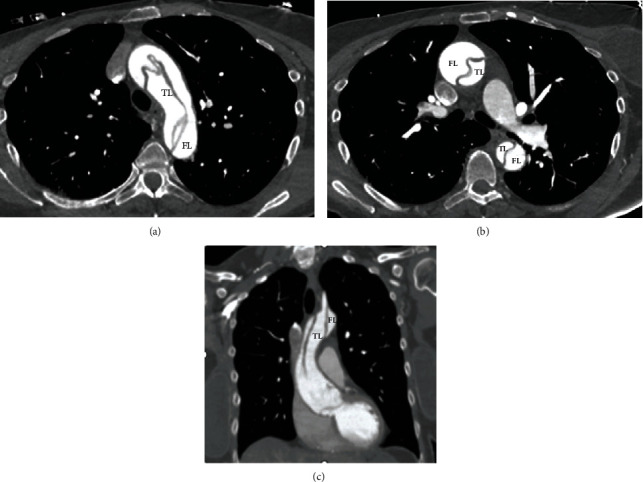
Computed tomography (CT) angiography showing aortic dissection in axial view (a, b) and dissection coronal view (c). FL: false lumen; TL: true lumen.
